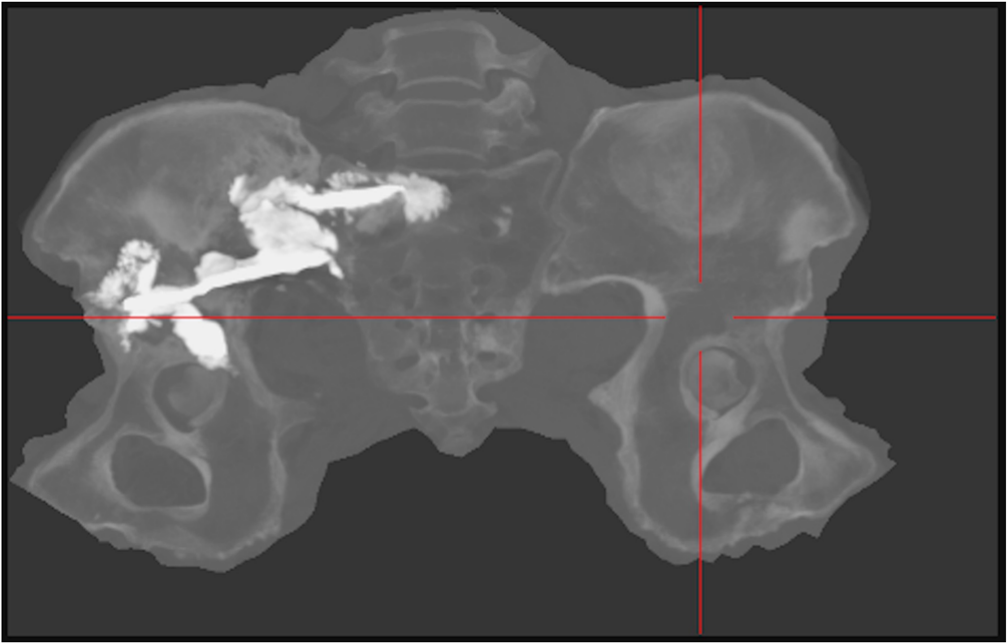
Postoperative CT-scan image with CT Bone Unfolding software representation showing position of the screws into the iliac and sacral bone and their bridging with cement.
